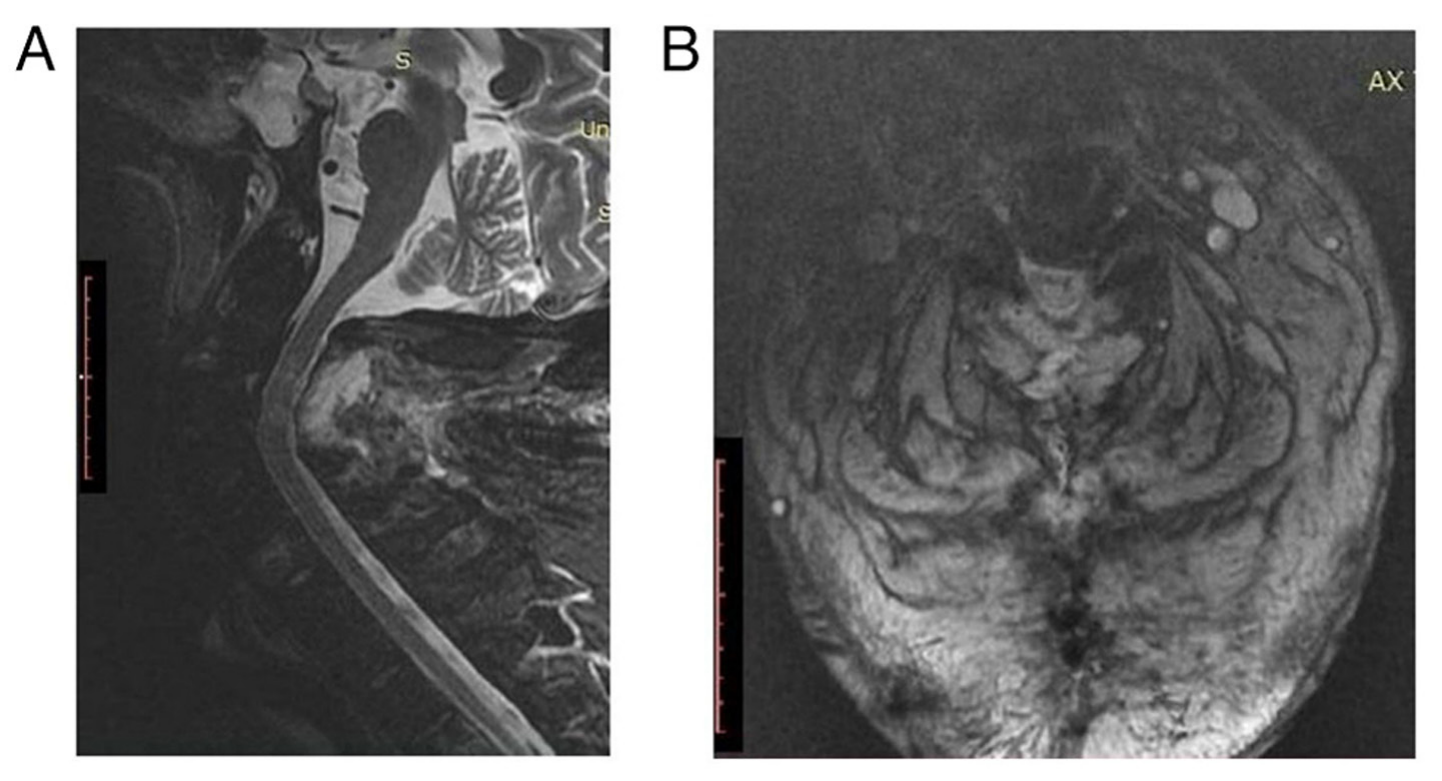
(A) Sagittal view and (B) axial view of a post-operative magnetic resonance imaging scan of a cervical spine documented the adequacy of decompression.
